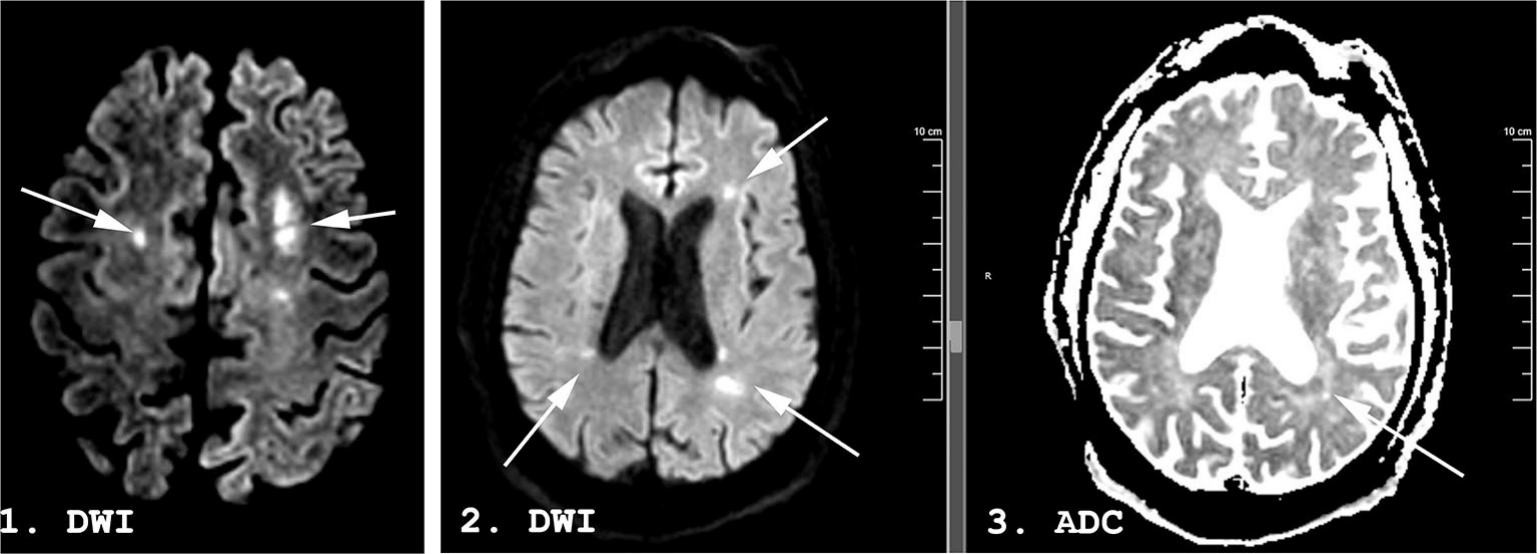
A 53-years-old man with COVID-19. Magnetic resonance imaging (MRI) performed 29 days following admission. Diffusion Weighted Imaging (DWI) in panels 1 and 2, apparent diffusion coefficient (ADC) in panel 3. Hyperintense lesions (arrows) are seen in the bilateral middle/anterior cerebral artery (MCA-ACA) borderzones on DWI. Some demonstrate a hypointense ADC correlate (arrow) while others are isointense on ADC, suggesting an acute to subacute timeframe.
